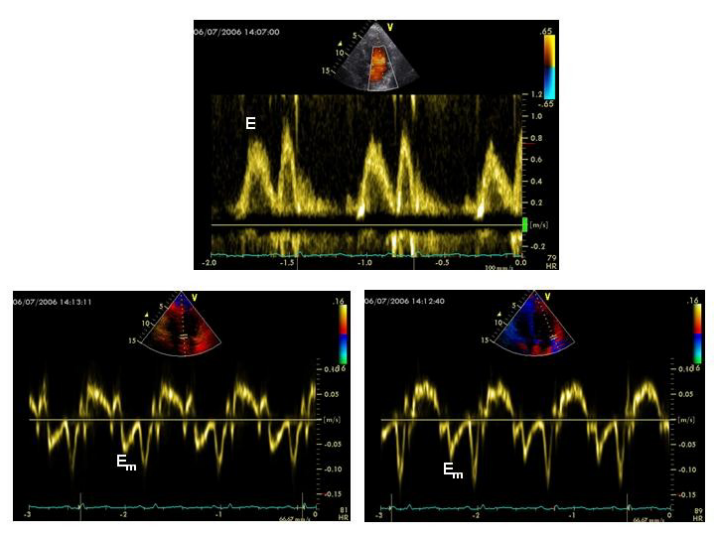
Sample of the combination of standard Doppler mitral inflow and Tissue Doppler of septal and lateral mitral annulus in a 38-year male patient affected by acute promyelocytic leukemia undergoing CT by idarubicine (12 mg/m^2^) + trans-retinoic acid (45 mg/m^2^). In the upper panel transmitral E/A ratio is > 1 (E peak velocity = 0.78 m/s); In the lower panels Tissue Doppler derived Em peak velocity is 0.07 m/s (left panel) and 0.08 m/s (right panel) respectively, determining an average Em = 0.075 m/s. Thus, E/Em ratio is = 10.4, indicating an initial increase of LV filling pressure (normal values of E/Em ratio < 8, see Ref # 90). E = transmitral early diastolic peak velocity, Em = Tissue Doppler derived myocardial early diastolic peak velocity.
